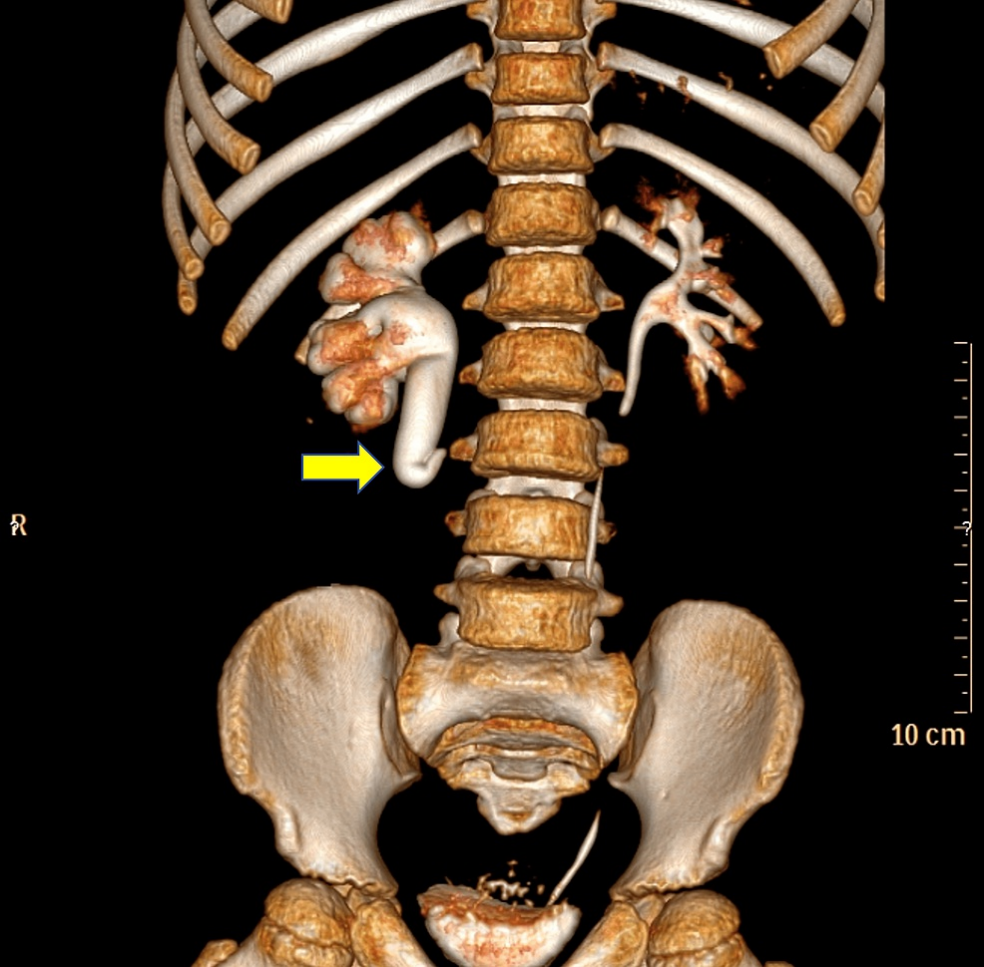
Three-dimensional computed tomography scan showing the right circumcaval ureter anomaly (yellow arrow) that encircles the inferior vena cava.
